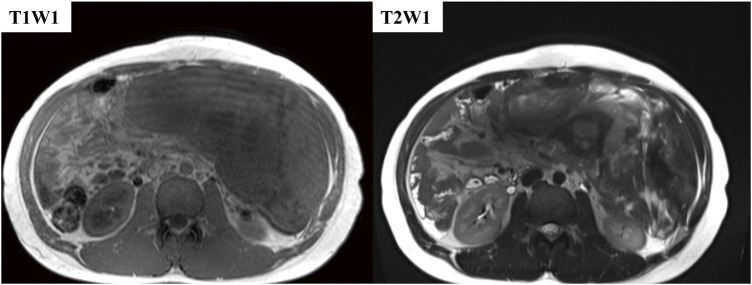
Abdominal MRI shows an intra-abdominal tumor measuring 34 × 15 × 8 cm with a low signal on T2W1, partially hyperintensity on T1W1, and isointensity on T1W1. No internal hemorrhage or fatty components are apparent. MRI, magnetic resonance imaging; T1W1, T1-weighted imaging; T2W1, T2-weighted imaging
